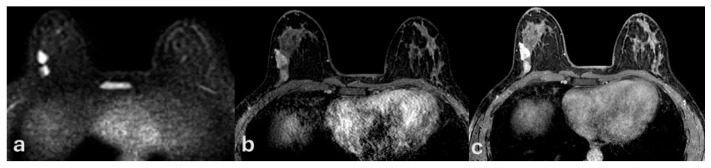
Contrast-enhanced MRI corespective for targeted breast ultrasound and digital mammography of patient of Figure 1. Contrast-enhanced dynamic MRI (a–c) depicts gradual enhancement without washout, consistent with a type II kinetic curve. Histopatology confirmed low-grade ductal carcinoma in situ (DCIS), despite the 2 cm radiological extension.
